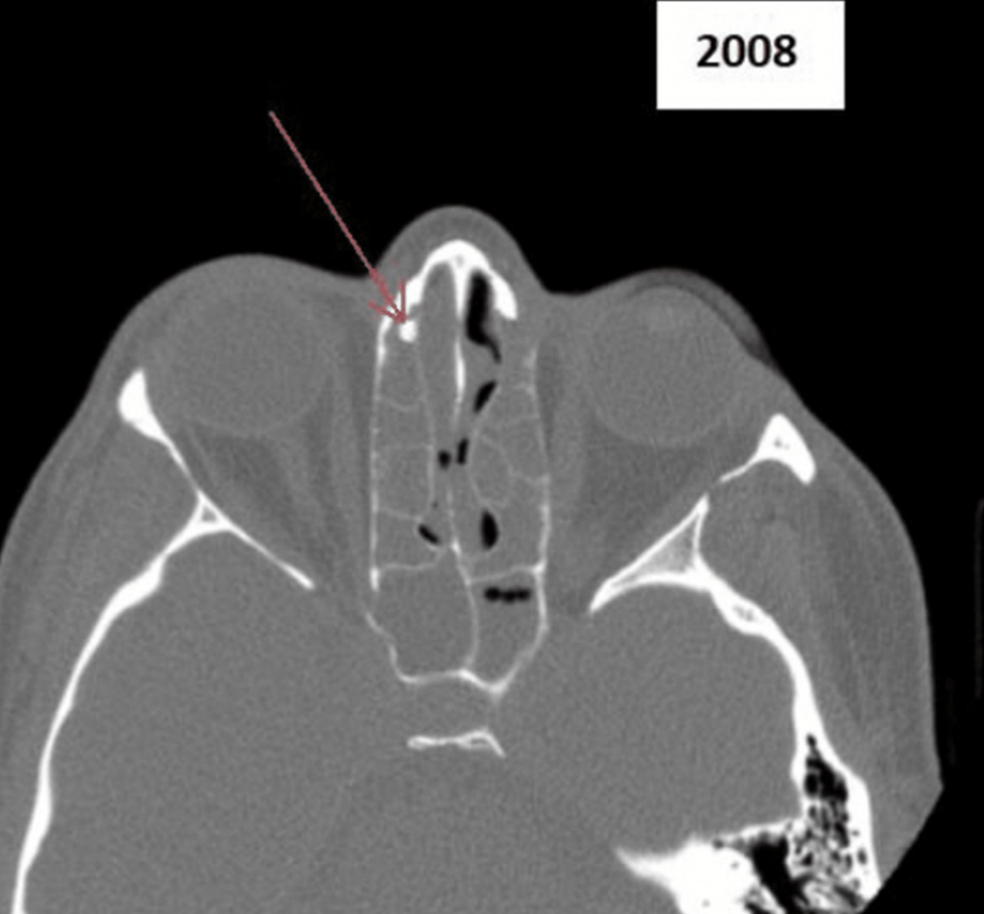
CT image of sinuses in 2008 The red arrow is showing the osteoma.
